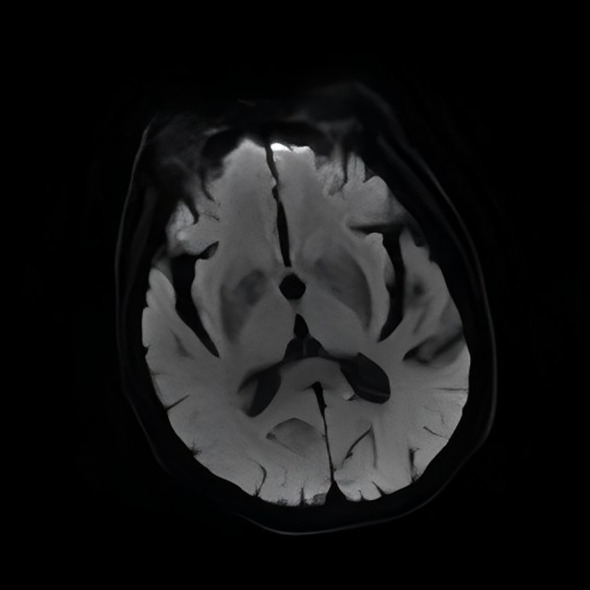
Diffusion-weighted imaging (DWI): No significant restricted diffusion in the brain parenchyma.
